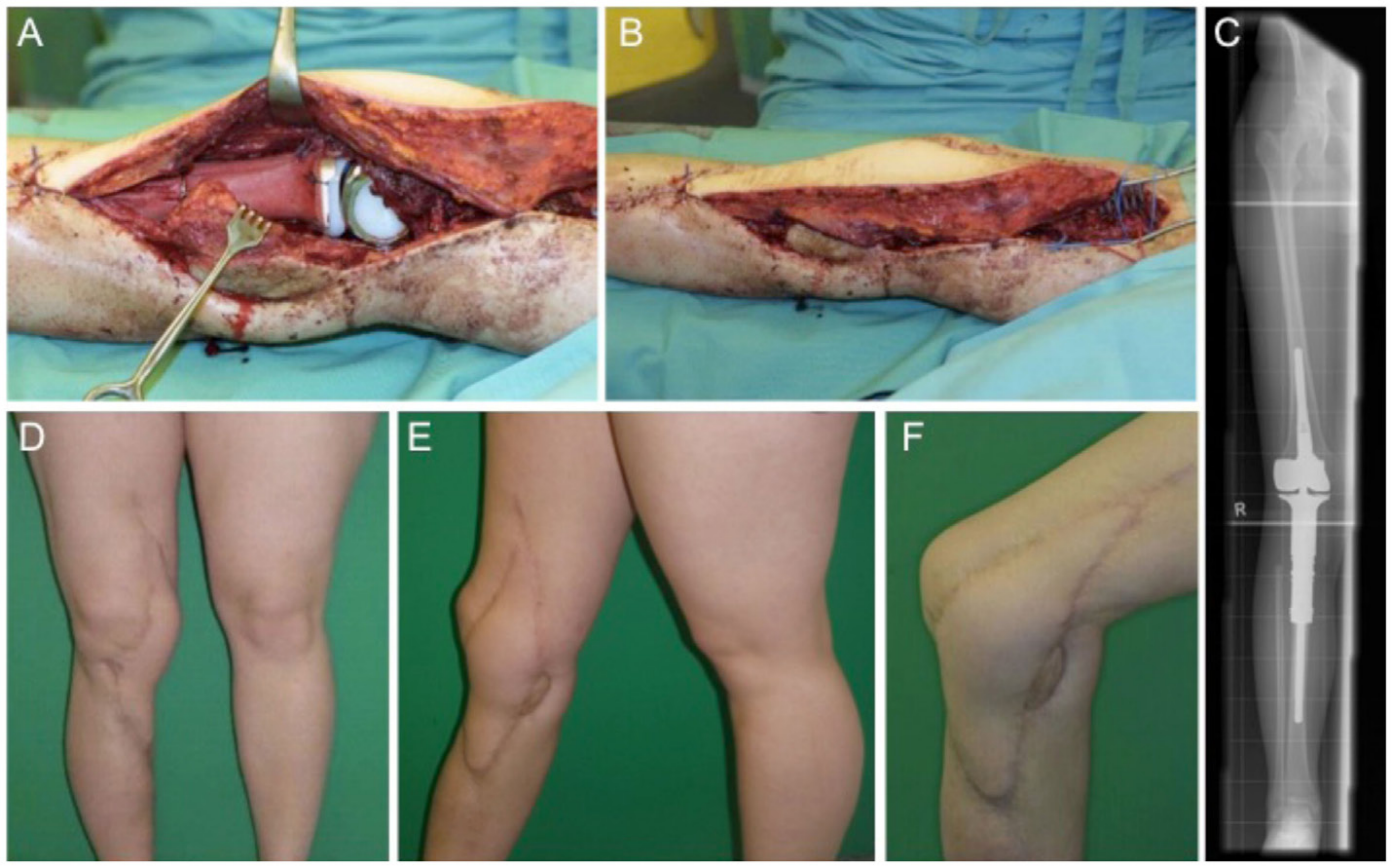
Intraoperative situs with implanted tumor prosthesis (MUTARS^®^) after extraarticular tumor resection of the knee joint (A,B) and coverage with a microvascular latissimus dorsi flap (B). (C) shows the corresponding x-ray image with a proximal tibial replacement. Post-operative esthetic and functional outcome (D–F).
